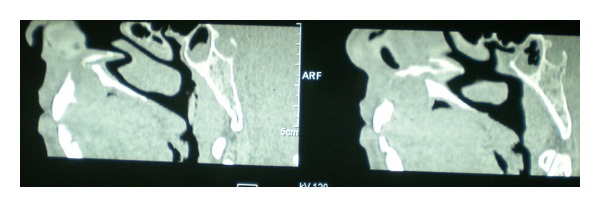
Sagittal sections of CT scan.
